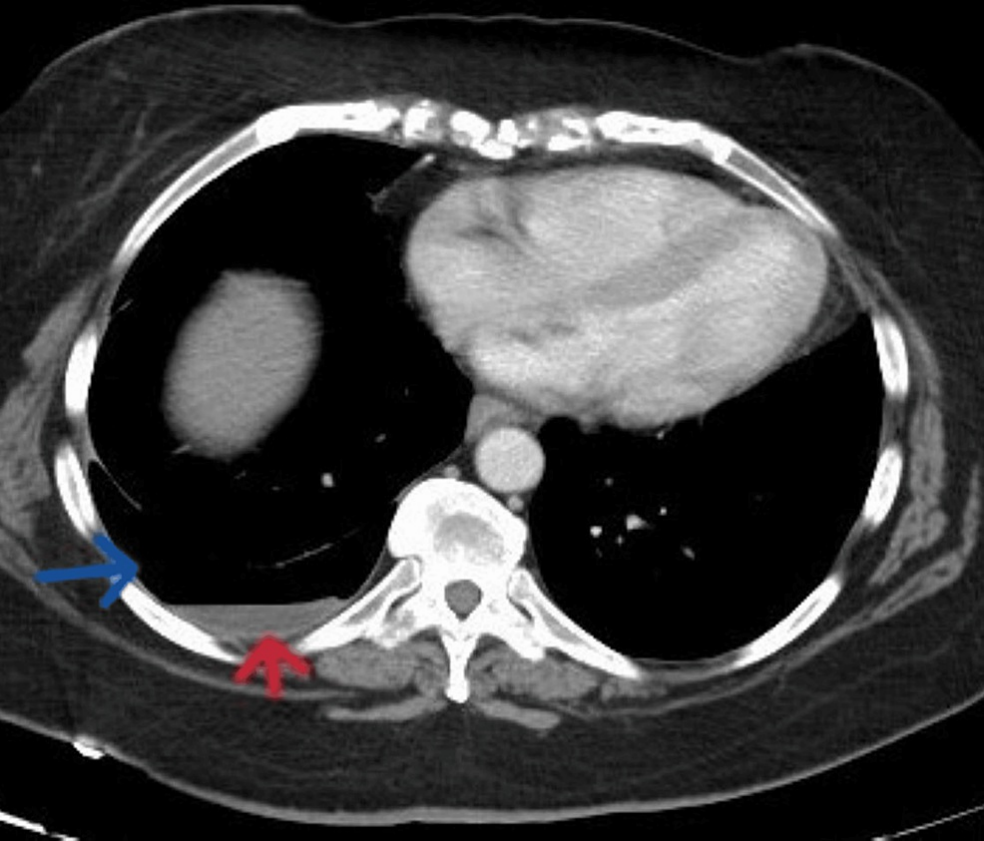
CT of the abdomen showing incidental right-sided hydropneumothorax (the red arrow shows hydrothorax and the blue arrow shows pneumothorax).
